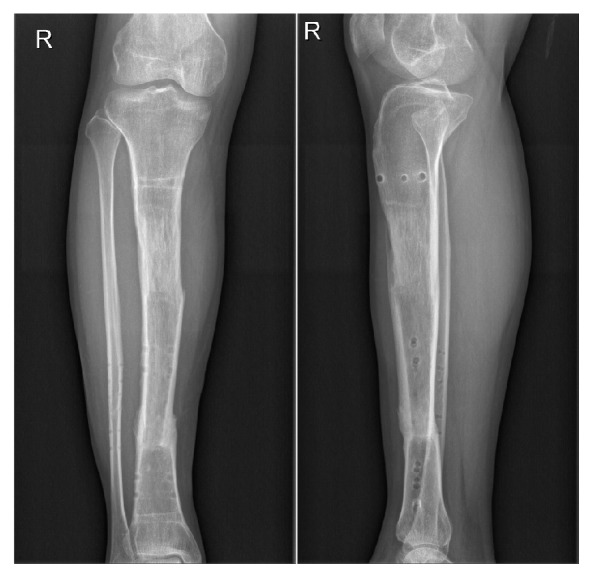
Anteroposterior and lateral X-ray photographs taken 3 months after the external fixation was removed.
